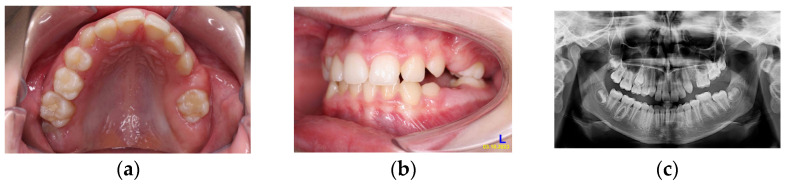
Age of 12 y and 11 m. Intraoral pictures (a,b) and panoramic X-rays (c) showed retained 26 and 27.
